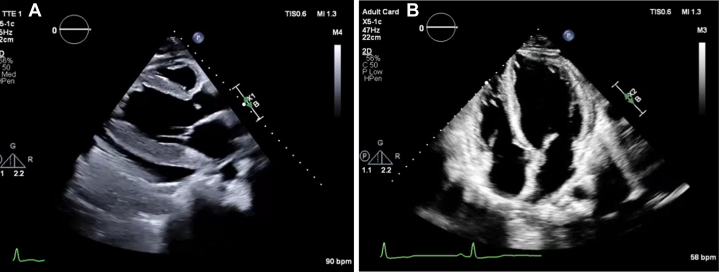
Transthoracic Echocardiography During Third Hospital Admission (A) Parasternal long-axis view showing moderate posterior and lateral pericardial effusion adjacent to the LV measuring 1.6 cm, with no RA/RV collapse. The LV is mildly dilated, LV systolic function is mildly decreased, EF is 44% ± 5%, with global hypokinesia of LV. The LVEF was visually estimated as indicating mild to moderate LV systolic dysfunction. LV diastolic function was indeterminate. (B) Apical 4-chamber view after treatment shows normal-sized LV. The LV systolic function was mildly decreased, EF was 50% ± 5%, LV diastolic function was indeterminate. The right ventricle was normal in size, RV systolic function was normal, and estimated RV systolic pressure was 22 mm Hg, consistent with normal pulmonary artery pressures. There is a small circumferential pericardial effusion measuring 0.6 cm predominantly anterior to the RV. EF = ejection fraction; LV = left ventricle; RA = right atrium; RV = right ventricle.
